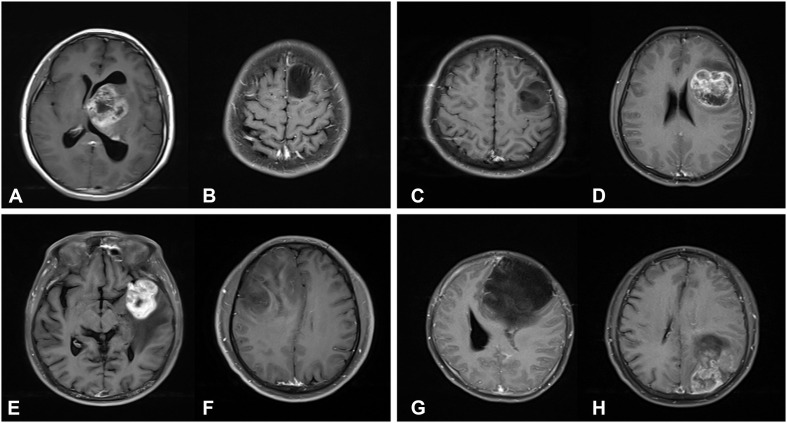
T1-weighted contrast-enhanced MR images. (A) A 23-year-old female patient with a grade IV glioma in left thalamus. The expression of S100β is strongly positive (S100β+++). (B) A 23-year-old male patient with a grade II glioma in left frontal lobe. The expression of S100β is weakly positive (S100β+). (C) A 27-year-old male patient with a grade II glioma in left frontal lobe. The expression of GFAP is strongly positive (GFAP+++). (D) A 27-year-old female patient with a grade IV glioma in left frontotemporal lobe. The expression of GFAP is weakly positive (GFAP+). (E) A 64-year-old male patient with a grade IV glioma in left frontotemporal lobe. The Ki67 index is 80%. (F) A 44-year-old male patient with a grade II glioma in right frontal lobe. The Ki67 index is 80%. (G) A 31-year-old female patient with a grade II glioma in left frontal lobe. Genetic test showed that IDH1 was mutant type. (H) A 50-year-old male patient with a grade IV glioma in left parietal-occipital lobe. Genetic test showed that IDH1 was wild type.
